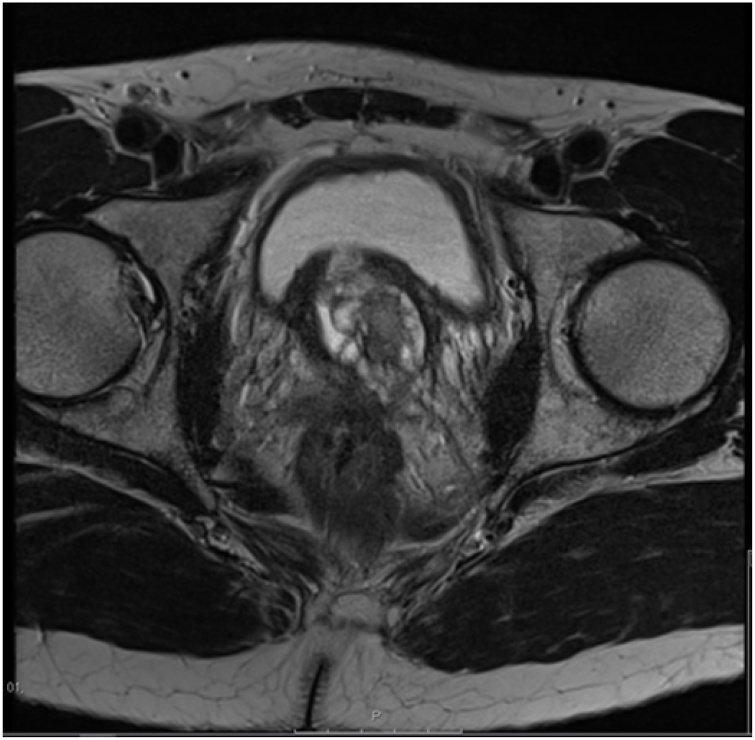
T2-weighted MRI scan (axial view) shows enlarged prostate growing into the bladder base pre-TURP.
